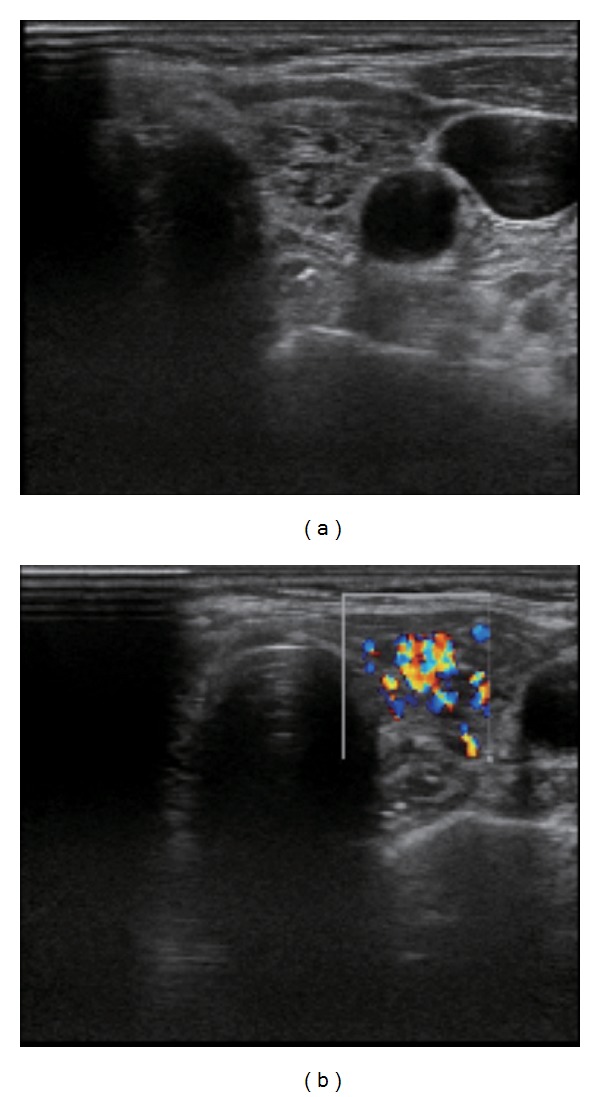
Ultrasonography of the left lobe. The remaining thyroid gland had an inhomogeneous appearance. There were no tumors in the left gland. Doppler color flow revealed a high vascularity in the remaining thyroid gland.
